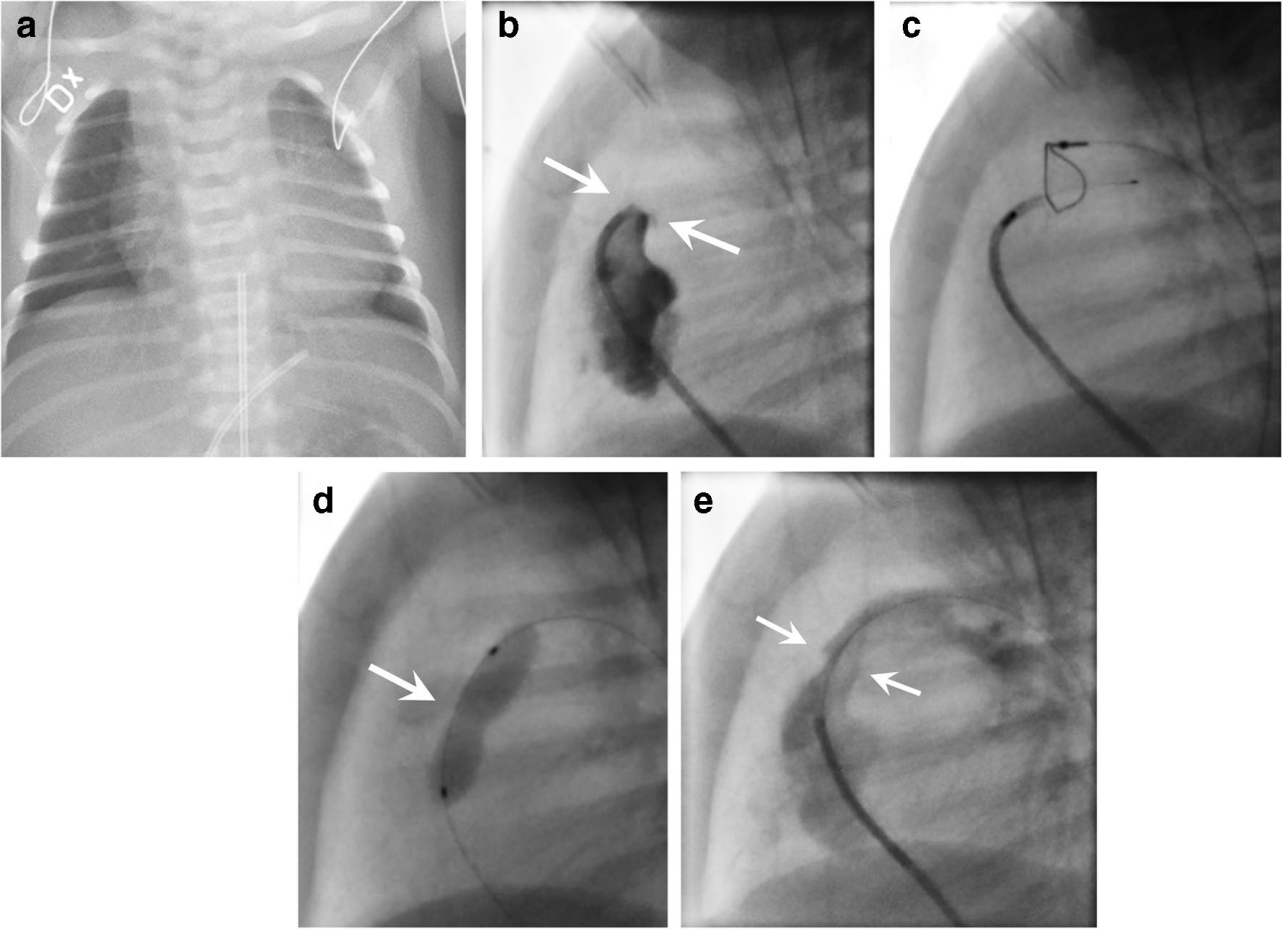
A newborn boy with a prenatal diagnosis of pulmonary atresia with intact ventricular septum. The diagnosis was confirmed by echocardiography after birth and prostaglandin infusion was started immediately to assure patency of the arterial duct. a Anteroposterior chest radiograph shows an enlarged heart and small size pulmonary vessels. Two umbilical catheters (one arterial and one venous are positioned in the midline subdiaphragmatically). b Lateral view from cardiac catheterization performed on day 3 of life shows the atretic pulmonary valve (*arrows*). c, d Treatment with radiofrequency perforation and a snare to open the valve (c) followed by balloon dilatation of the valve (*arrow in*
